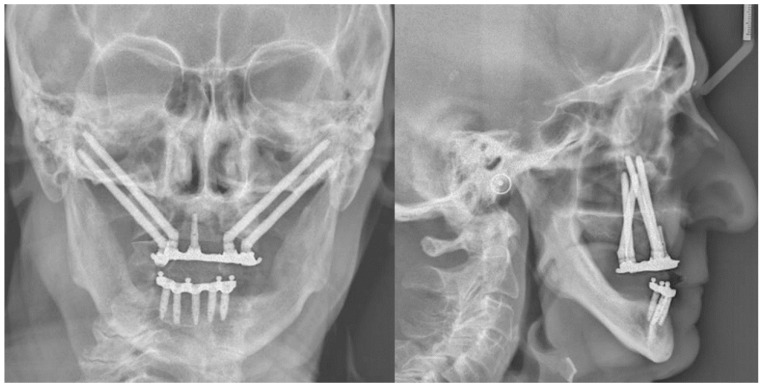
Postoperative frontal and lateral radiographs.
